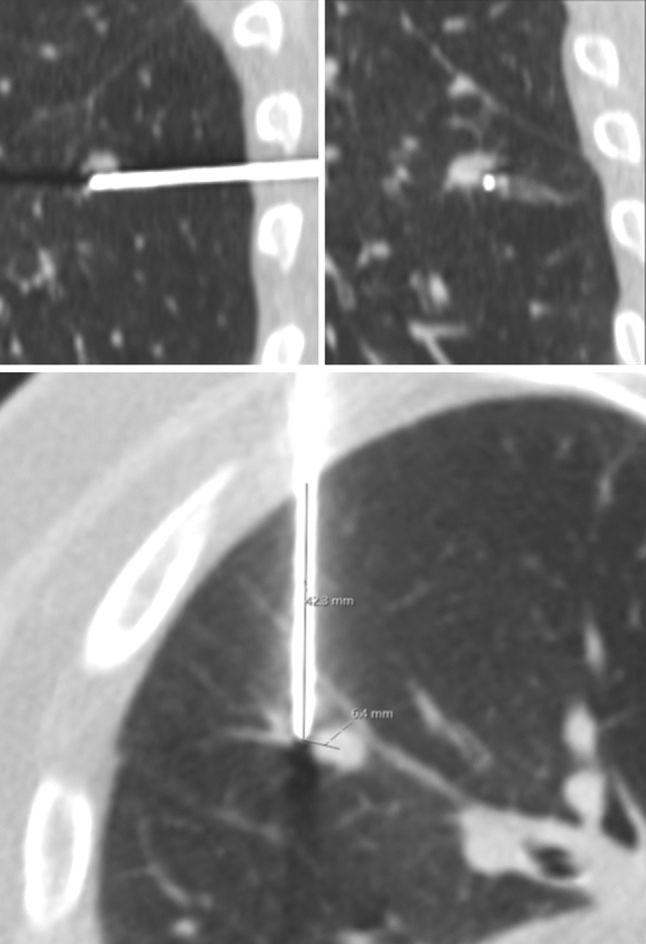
Unenhanced multiplanar reformat CT images of an 85-year-old man undergoing ablation of a left lower lobe sigmoid cancer lung metastasis. Images show measurement of first co-axial needle placement tip-to-target distance of 6.4 mm, and a parenchymal transgression length of 42 mm. N.B. This degree of error may be tolerable for satisfactory ablation due to array diameter of 3 cm or greater
